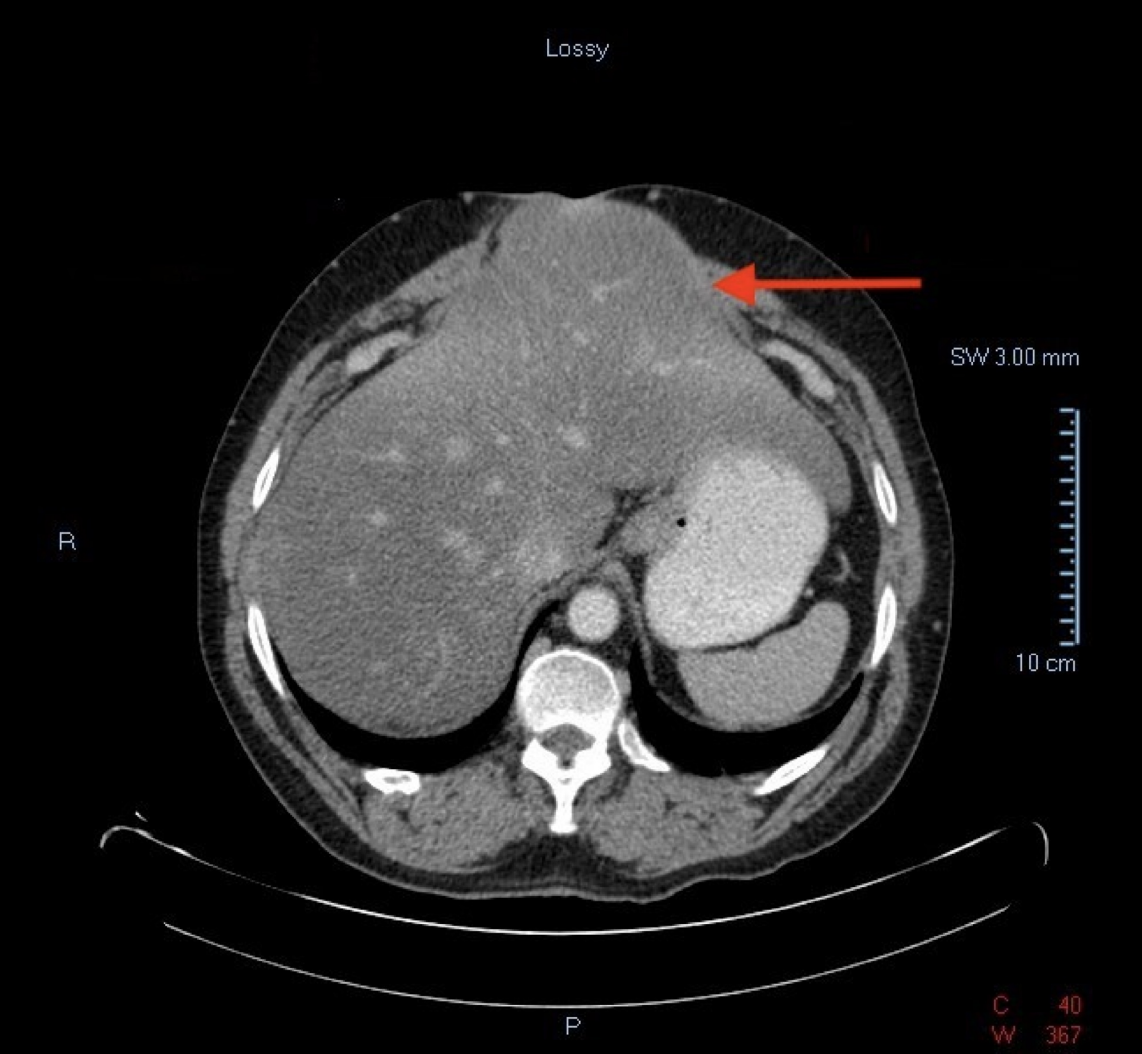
CT abdomen (axial view) showing herniation of the left hepatic lobe through an incisional abdominal hernia.
